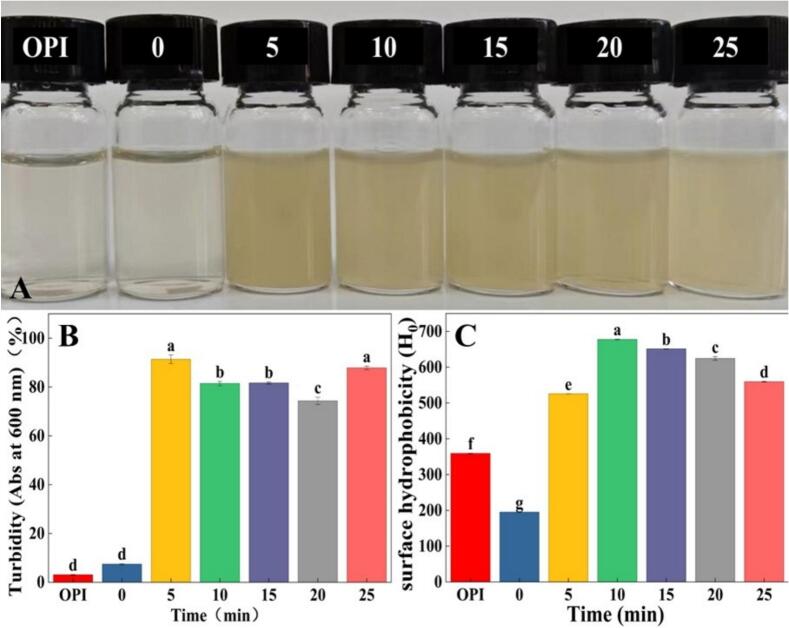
(A) Visual appearance, (B) turbidity, and (C) surface hydrophobicity of OPF subjected to different ultrasonic treatment times (0, 5, 10, 15, 20, 25 min) and untreated OPI. Different letters indicate significant differences (*P* < 0.05).
